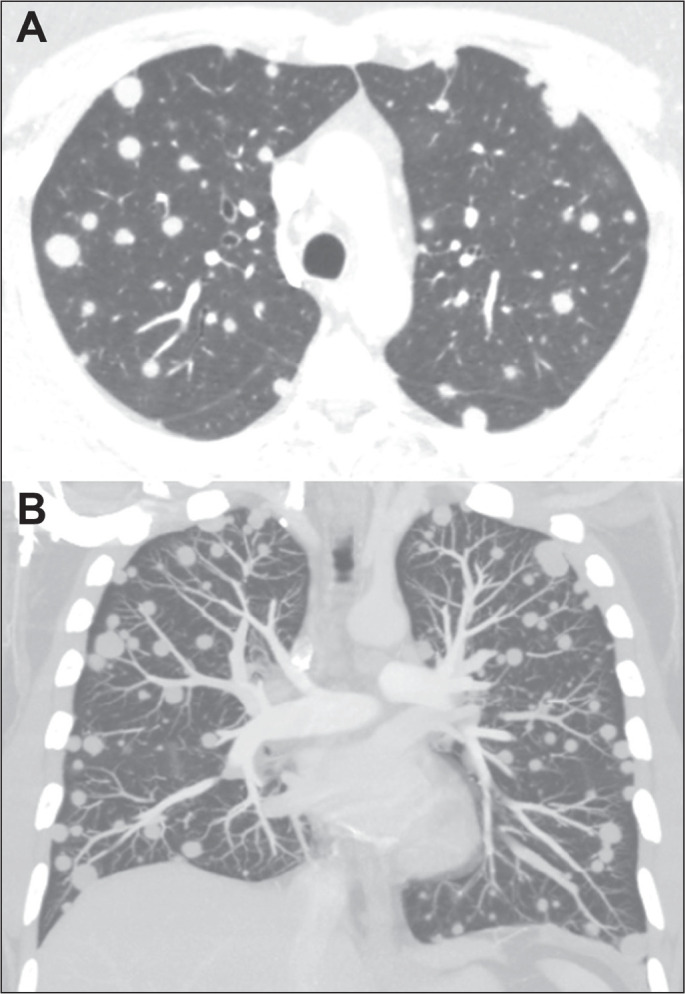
CT of a 40-year-old man diagnosed with pulmonary histoplasmosis by
